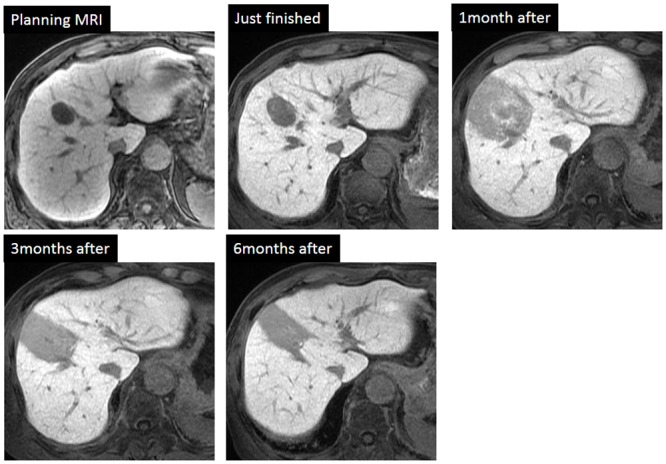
Gd-EOB-DTPA MRI scans showing changes in the irradiated HCC and the background liver after PBT. On the follow-up Gd-EOB-DTPA MRI scan, FLR did not appear 1 month later in this case. The contours of the FLR were made manually on MR images at 3 or 6 months after PBT. The dFLR contour (50% isodose line in this case) is defined as the area irradiated at doses exceeding the TD at planning MRI and gradually shrinks to the FLR area at 3 or 6 months after PBT. HCC, white arrow head; dFLR contour, yellow; FLR contour, green line. Abbreviations; Gd-EOB-DTPA MRI: Gadolinium ethoxybenzyl diethylenetriamine pentaacetic acid-enhanced magnetic resonance imaging; HCC: Hepatocellular carcinoma; PBT: Proton beam therapy; FLR: Focal liver reaction; destined FLR area: dFLR; TD: Threshold dose.
